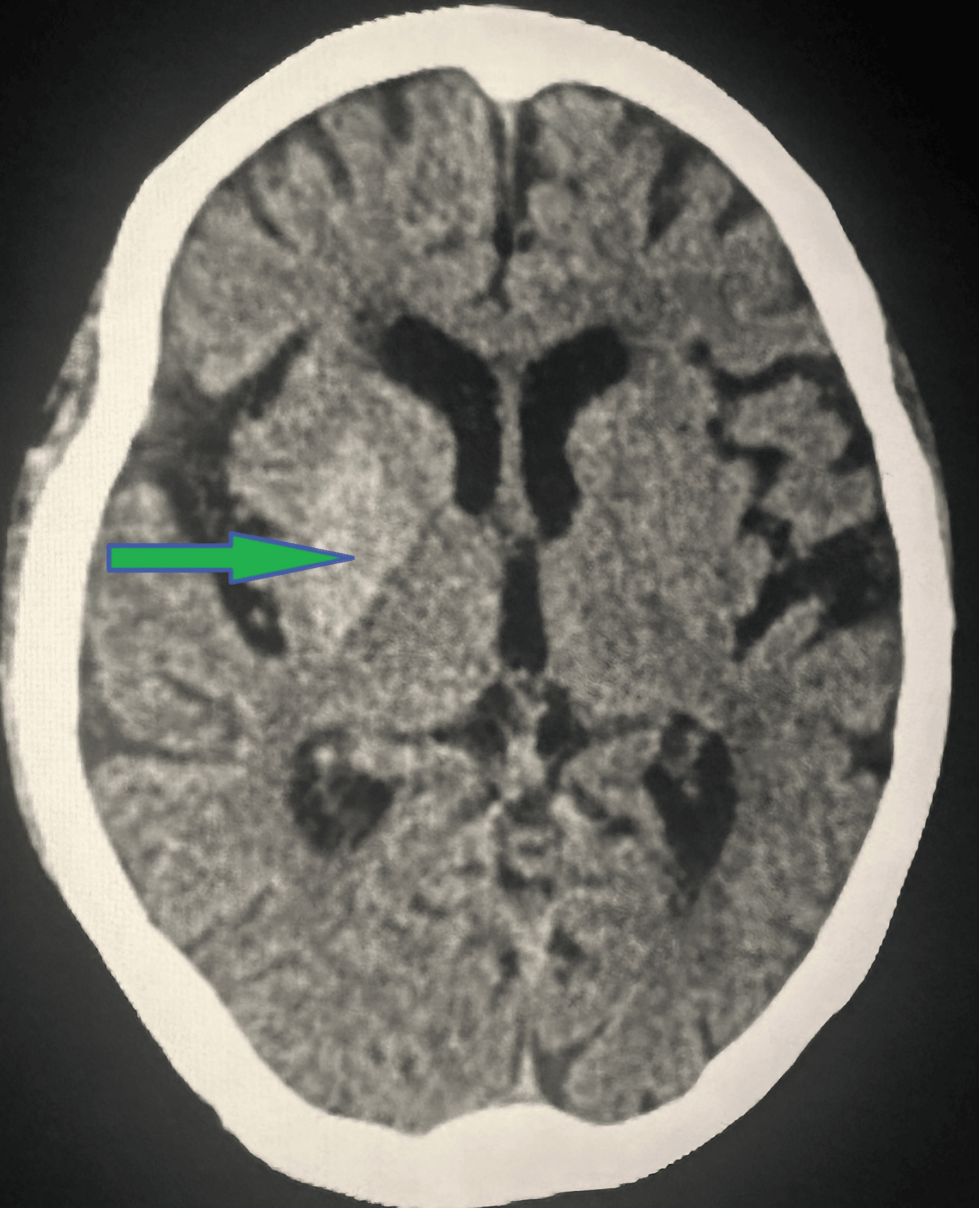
CT brain findings The red arrow shows hyperdensity of HU +35 to +43 noted in the right lentiform nucleus-features suggestive of non-ketotic hyperglycemic hemichorea. CT: Computed tomography, HU: Hounsfield unit
